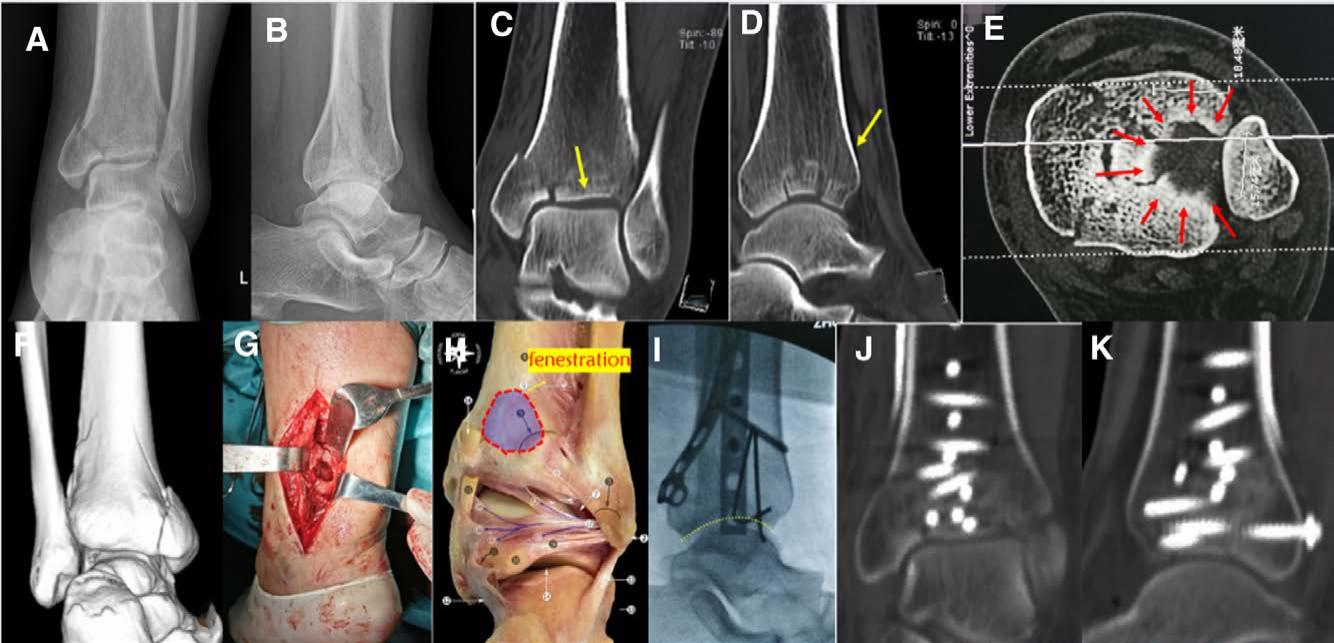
Frontal and lateral X-rays of the left ankle upon admission to the hospital (A and B). CT scan revealed a comminuted fracture involving the inner ankle, central region, and posterior lateral aspect of the distal tibia, with intact anterolateral bone cortex (C–F). Intraoperative windowing beyond the posterior articular surface of the distal tibia (G and H). The distal articular surface of the tibia was well reduced after posterior tibial fenestration indirect reduction and compression (I). The postoperative CT scan revealed satisfactory reduction of the articular surface, with central collapse (J and K). CT = computed tomography.
